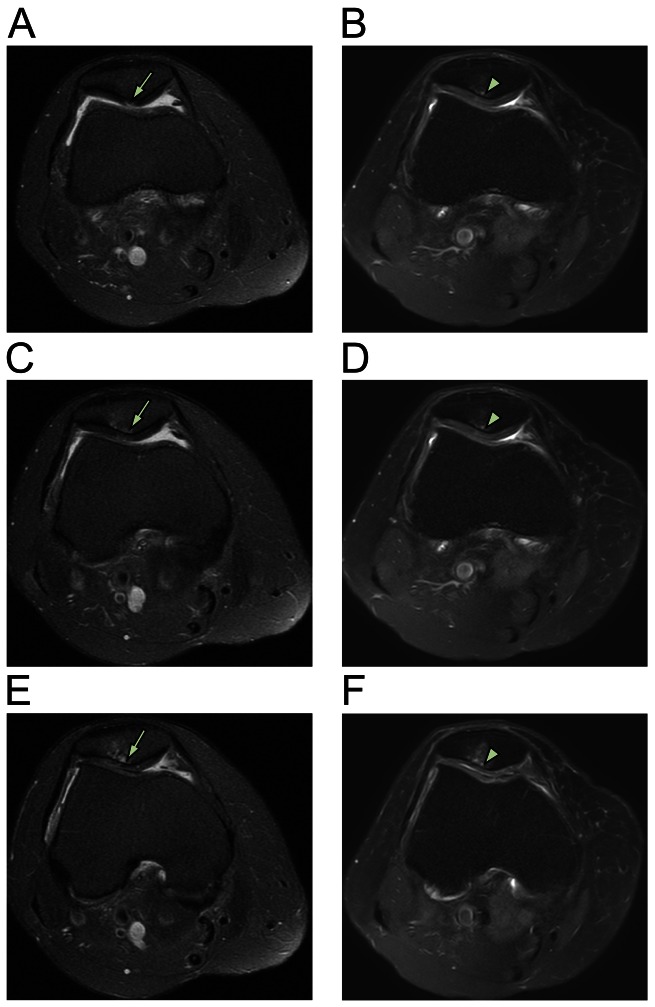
MRI axial sequential T2 views patient 3. Pre-treatment MRI scans of patient 3 (A [4/19], C [5/19], and E [6/19]) show retro-patellar signal changes (arrow) consistent with chondromalacia patellae along with medial meniscal maceration and cartilage thinning consistent with osteoarthritis. Post-treatment MRI scans at three months (B [5/20], D [6/20], and F [7/20]) show changes (arrowhead) consistent with probable cartilage restoration.
